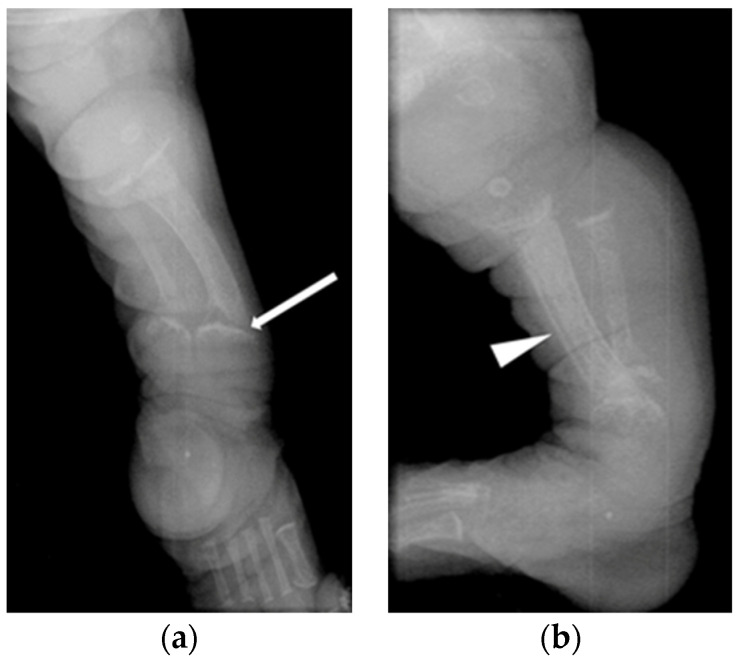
Acute findings of hyperparathyroidism and of rickets. Patient 2, right leg radiograph day 4 (a) frontal and (b) lateral views show features of hyperparathyroidism (best seen on the lateral view) including reduced bone density, subperiosteal resorption and poor cortical delineation as well as diaphyseal cloaking of the tibia (arrowheads). Features of rickets are seen with cupped, splayed and frayed metaphyses in all the bones. A submetaphyseal lucent band is seen in the tibia (white arrow). Talocalcaneal stippling is also present.
